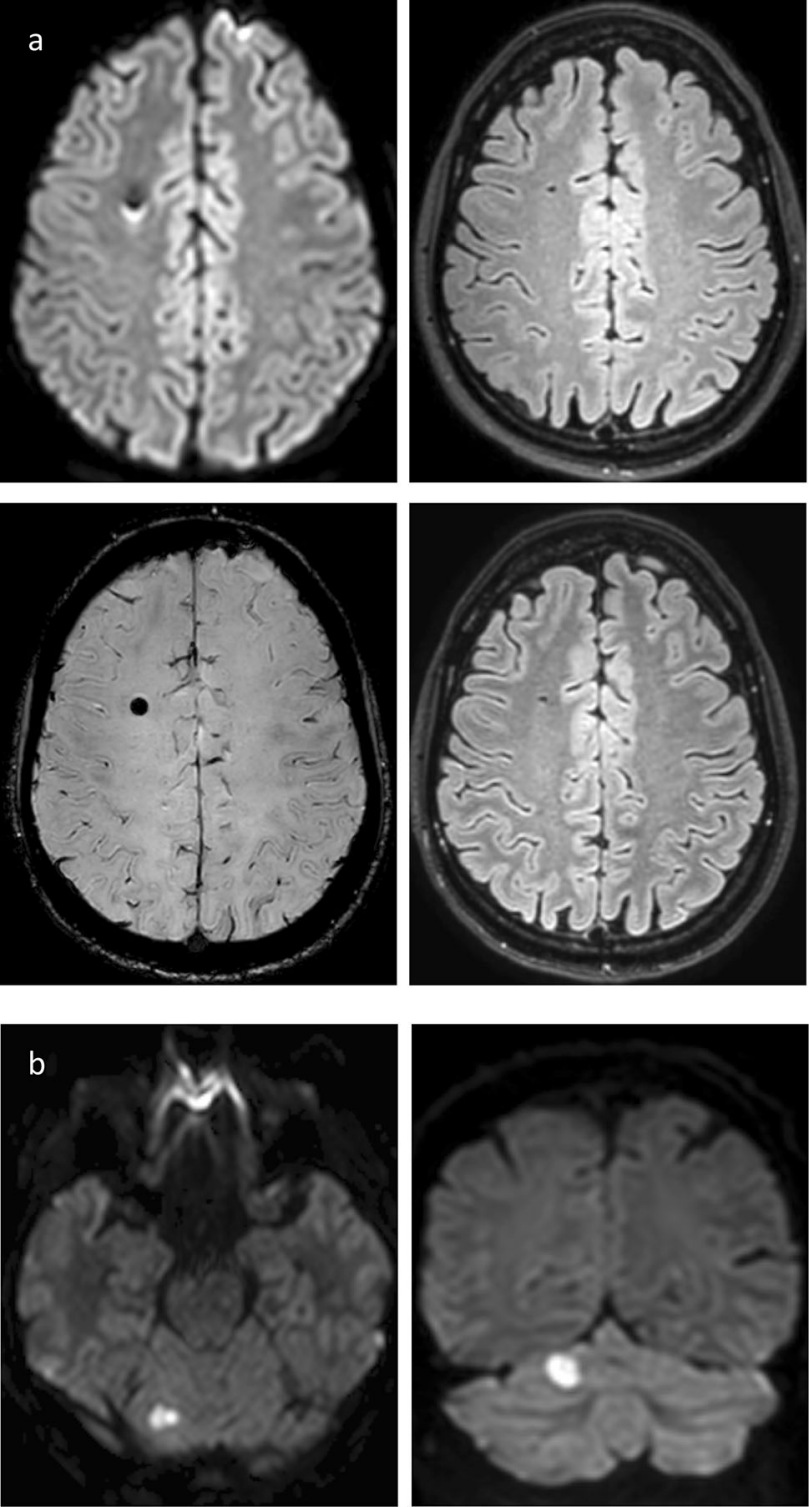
Atypical appearance of intracranial lesions following diagnostic DSA. a Subject No. 4. Upper row shows DWI (left) and FLAIR (right) immediately after DSA. Bottom row shows SWI (left) and FLAIR (right) at follow-up appointment. A lesion of central low DWI signal and a bright posterior rim with corresponding low signal in FLAIR can be seen after DSA. Follow-up shows high susceptibility with blooming in SWI and predominantly unchanged characteristics in FLAIR 7.7 months after DSA. b Subject No. 76. Axial DWI (left) and coronary DWI (right) after DSA show a roundish and unusually large DWI bright diffusion-restricted lesion in the right upper cerebellar lobe. Subject was lost to follow-up. FLAIR, Fluid attenuated inversion recovery. DSA, Digital subtraction analysis. DWI, Diffusion-weighted imaging. No, Number. SWI, Susceptibility-weighted imaging
